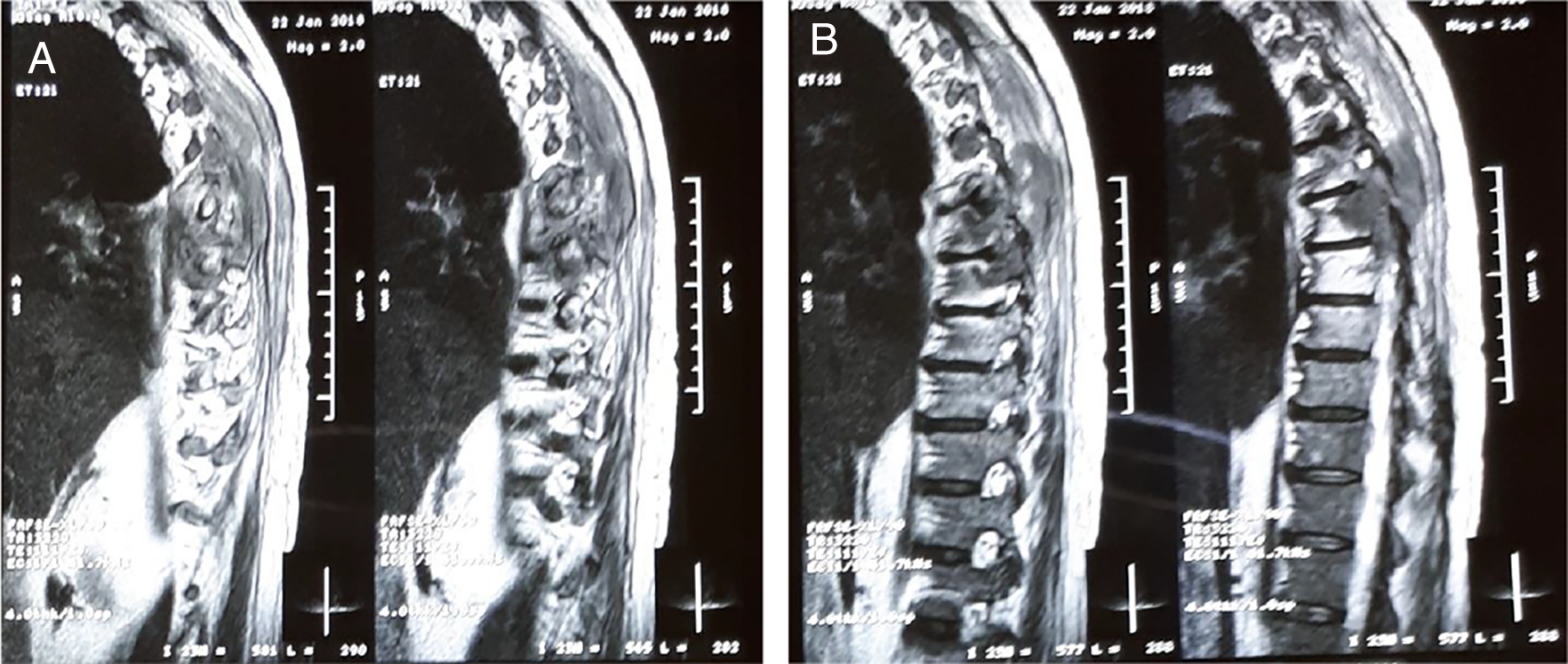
Magnetic resonance imaging (MRI) imaging of thoracic vertebra depicting tumour invasion on T6–T8 vertebra and into the root canal with resulting pressure into the spinal cord.
